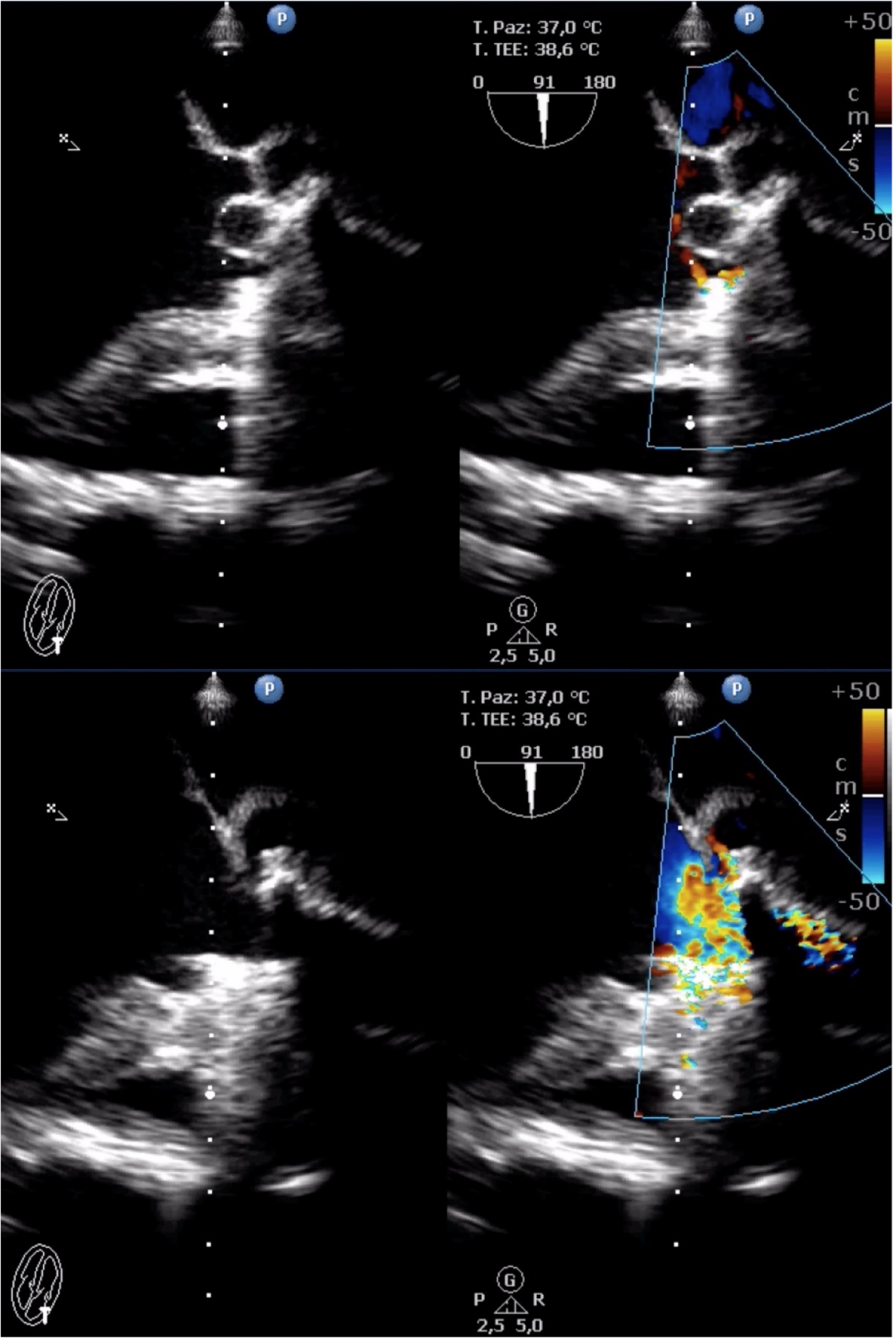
Transthoracic echocardiogram showing a hypoanechoic formation at the level of the aortic root in the periprosthetic area, which extended from the subannular plane into the periprosthetic site with apparent compressive effect on the first tract of the ascending aorta
